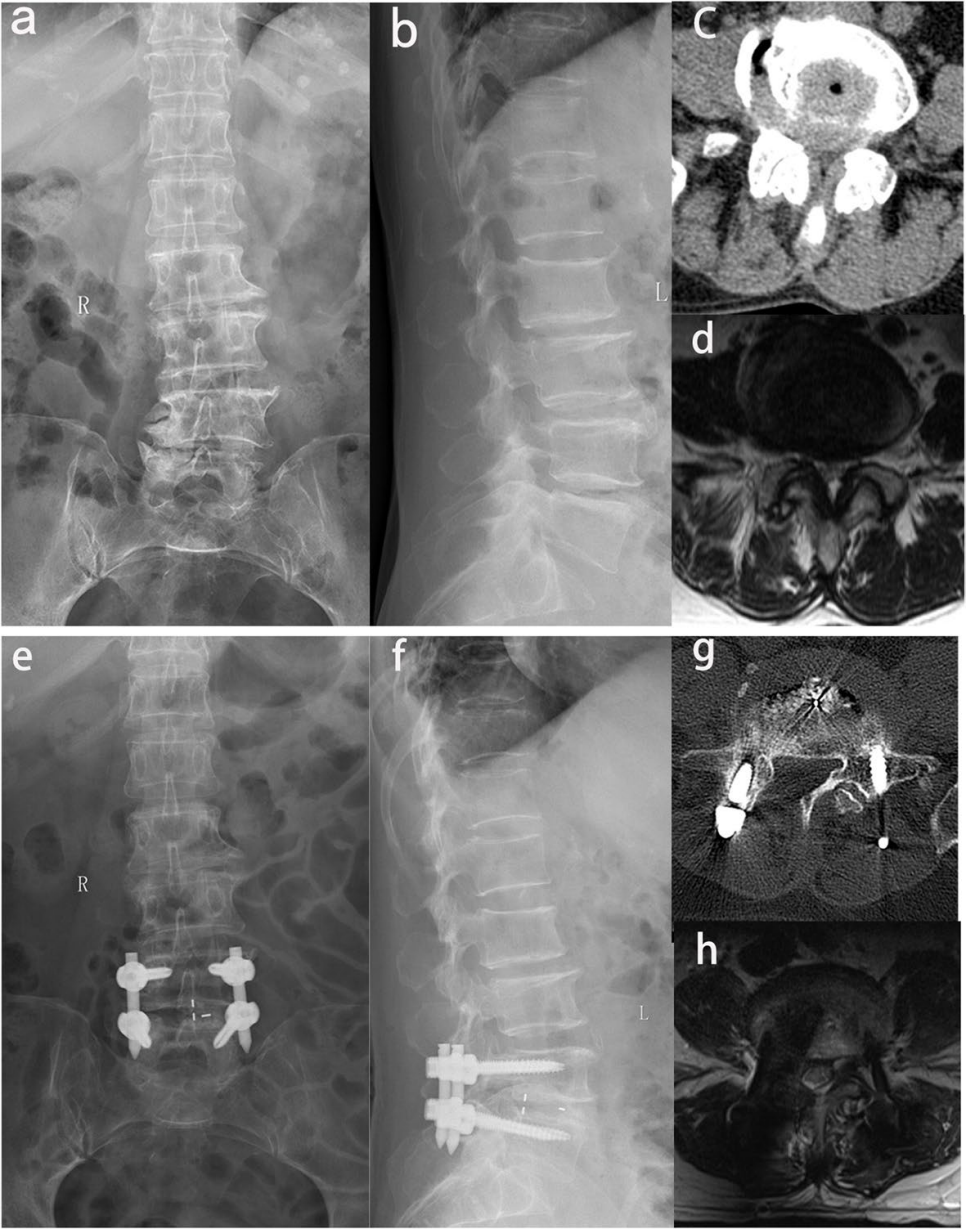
In the group of ultrasonic bone curette, a 74-year-old woman suffered from low back pain accompanied by intermittent claudication for more than three years. Preoperative X-ray (a, b), MRI (d), and CT (c) examinations showed severe DLSS (Schizas Grade D) at the L4/L5 level. The patient received Mis-TLIF assisted by an ultrasonic bone curette, and symptoms were significantly relieved after the surgery. Postoperative X-ray (e, f) and postoperative CT/MRI indicated that complete decompression was achieved at L4/L5 (g, h)
